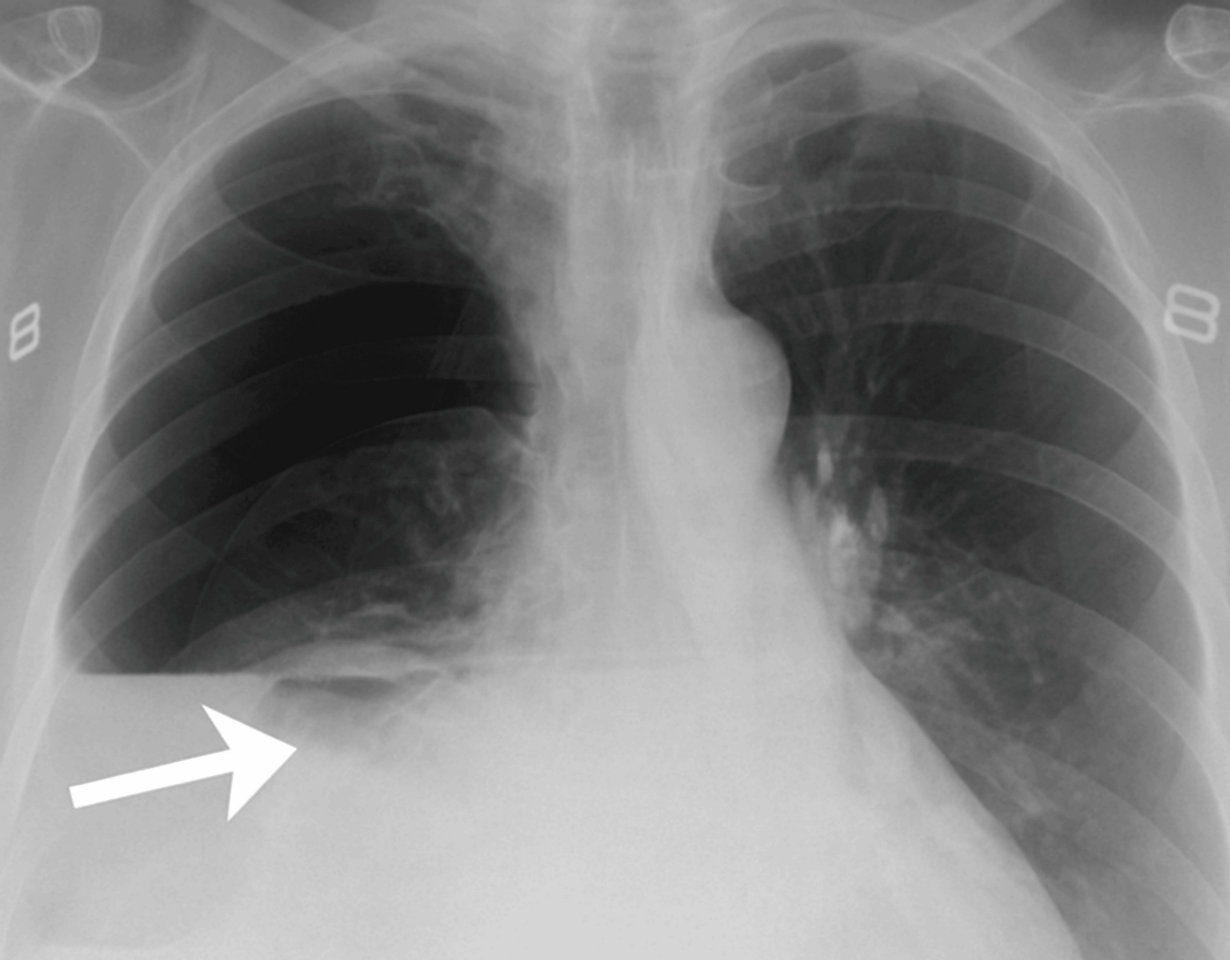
Chest X-ray (frontal view) showing a large right-sided radiolucent area with a visible air-fluid level, suggestive of hydropneumothorax. There is significant opacity occupying the lower right hemithorax, with a sharp horizontal margin indicating a fluid level. The left lung appears fully expanded. A prominent arrow highlights a region near the right hemidiaphragm, where subtle curvilinear densities—possibly representing bowel loops—are faintly visible but were initially overlooked. This image was misinterpreted as a large hydropneumothorax, leading to chest drain insertion before a CT scan confirmed the actual diagnosis of a right-sided diaphragmatic hernia.
